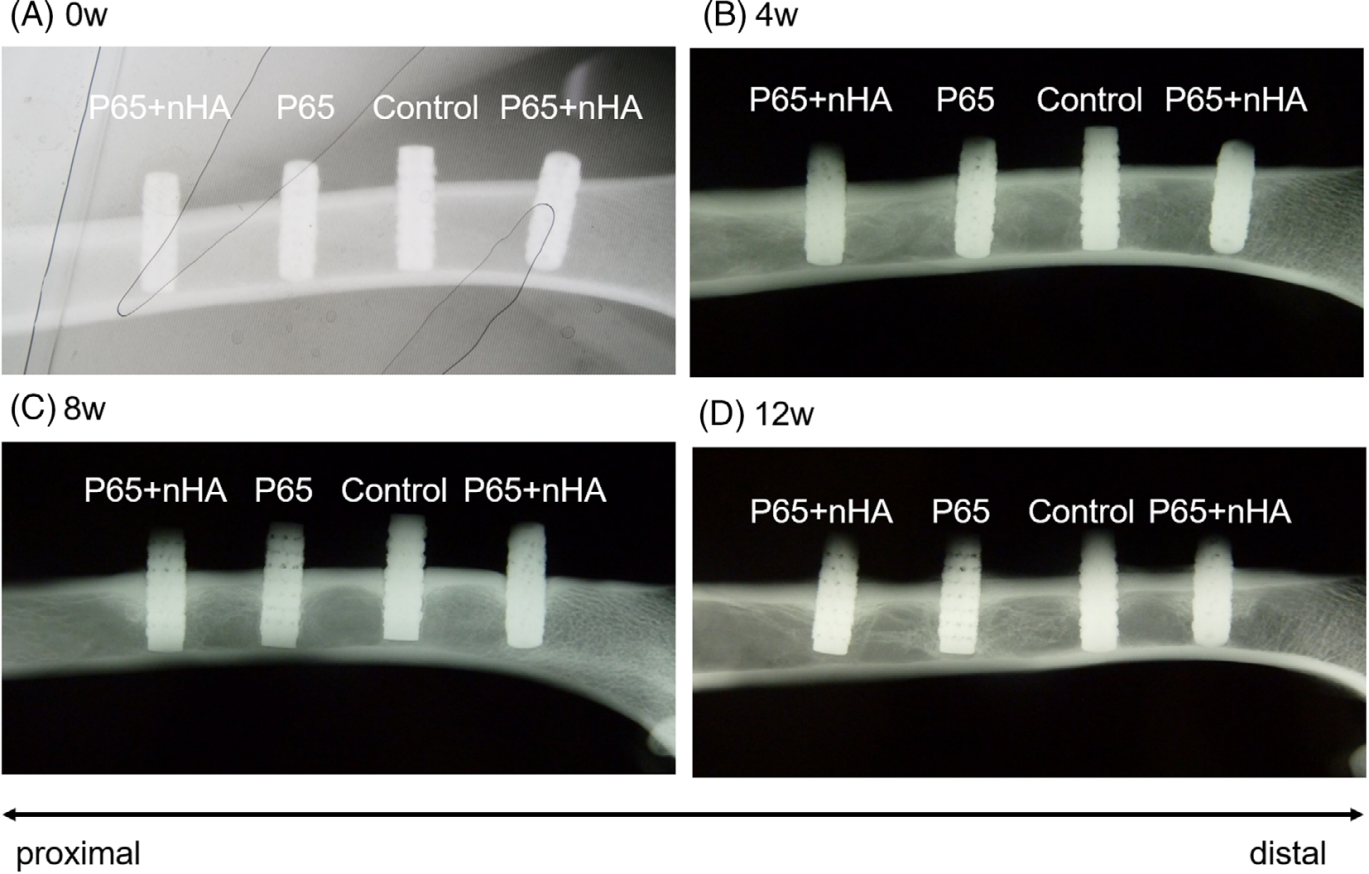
Radiographs of femurs harvested at 0, 4, 8, and 12 weeks postoperatively showing extensive peri‐implant bone formation around all implants
